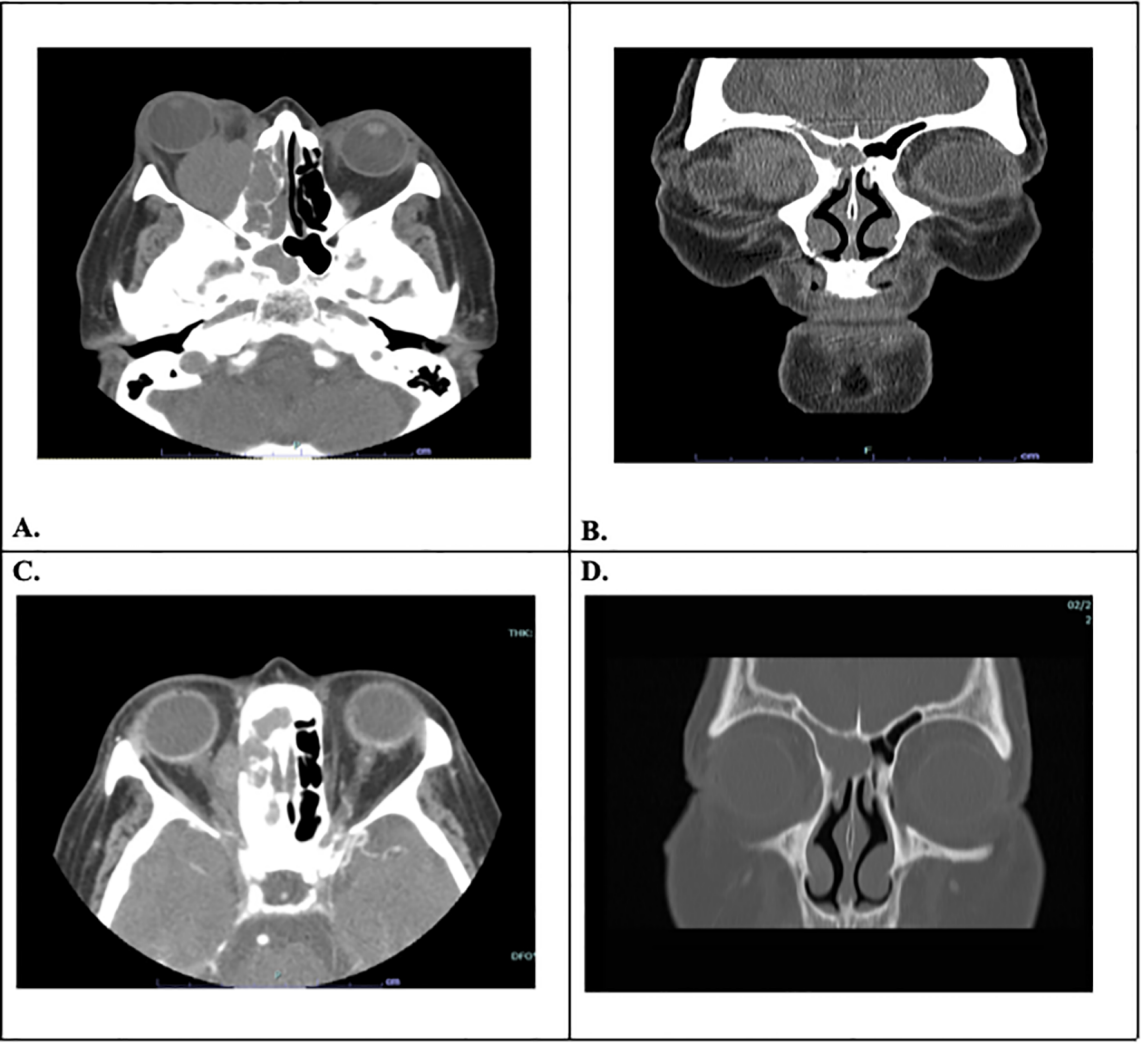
(A) Axial high-resolution CT showing orbital infiltration with right optic nerve compression. (B) Coronal high-resolution CT showing orbital infiltration. (C) Axial high-resolution 1-month post-operative CT. (D) Coronal high-resolution 1-month post-operative CT.
